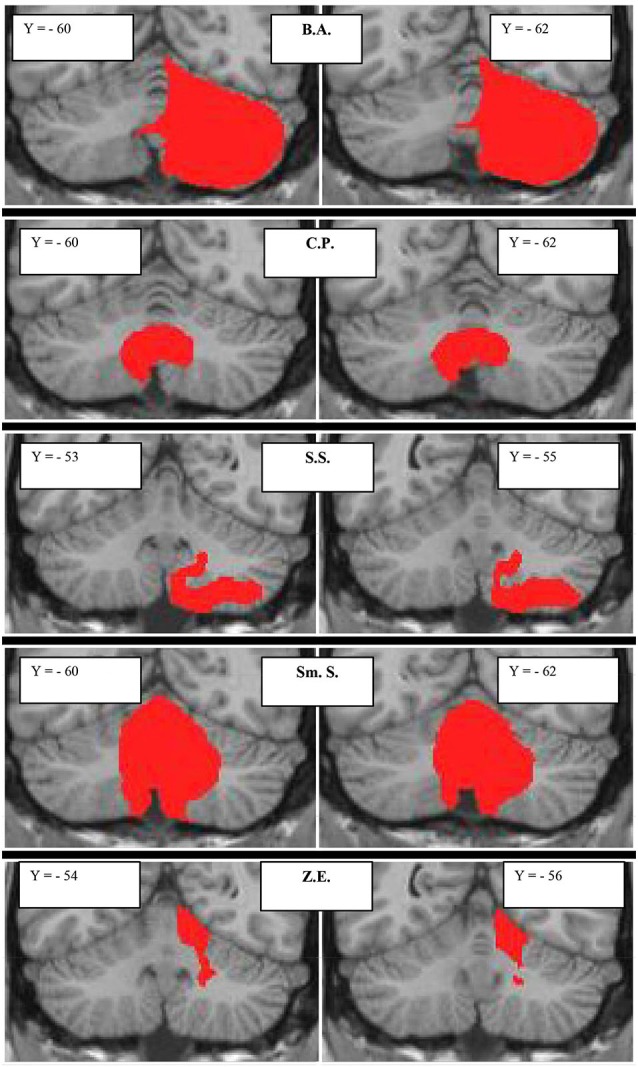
Subjects with focal cerebellar lesions. Lesion extensions were assessed on 3D-T1-MPRAGEs after spatial normalization and overlaid onto a coronal T1-weighted template from Schmahmann et al. (2000). For each subject, the lesion is shown in two representative coronal sections. The case code is as in Table 2.
